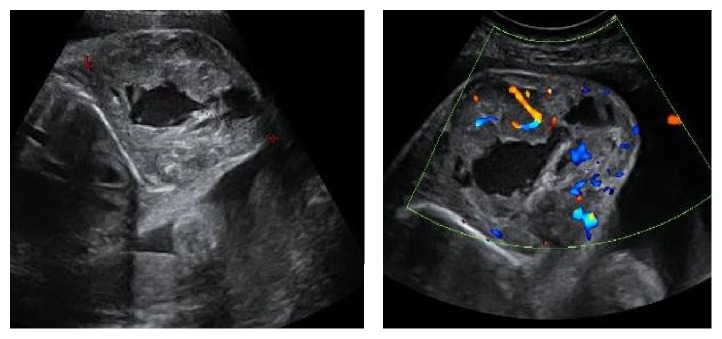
Fetal ultrasound showing the tumor in the left armpit.
